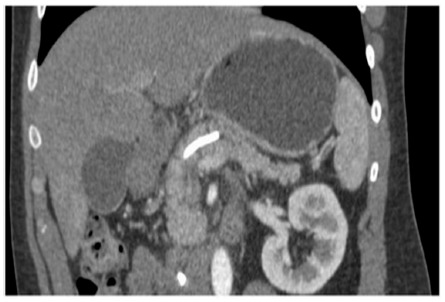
Abdominal CT showed complete resolution of the pancreatic fluid collection
